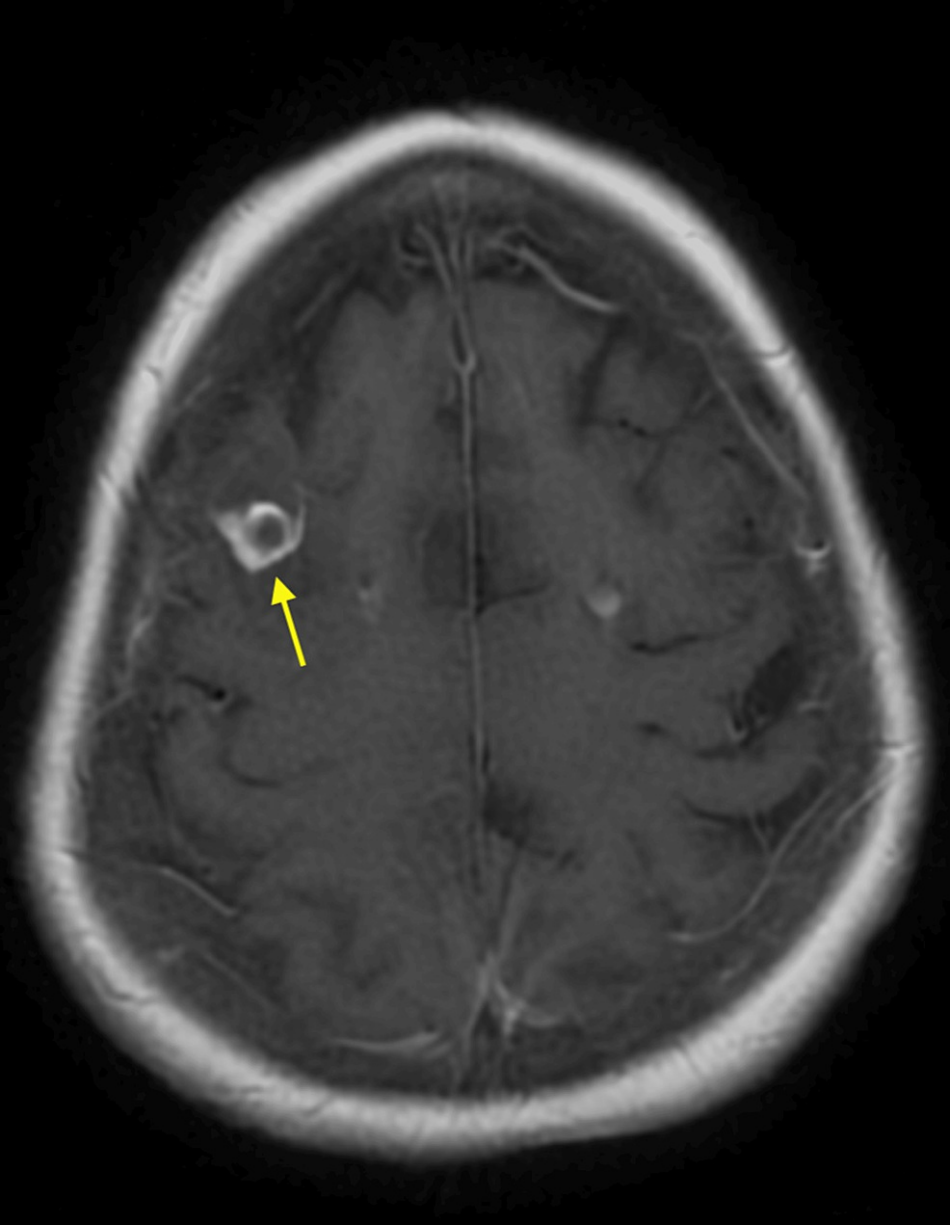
Magnetic resonance imaging (MRI) showing a ring enhancing lesion
